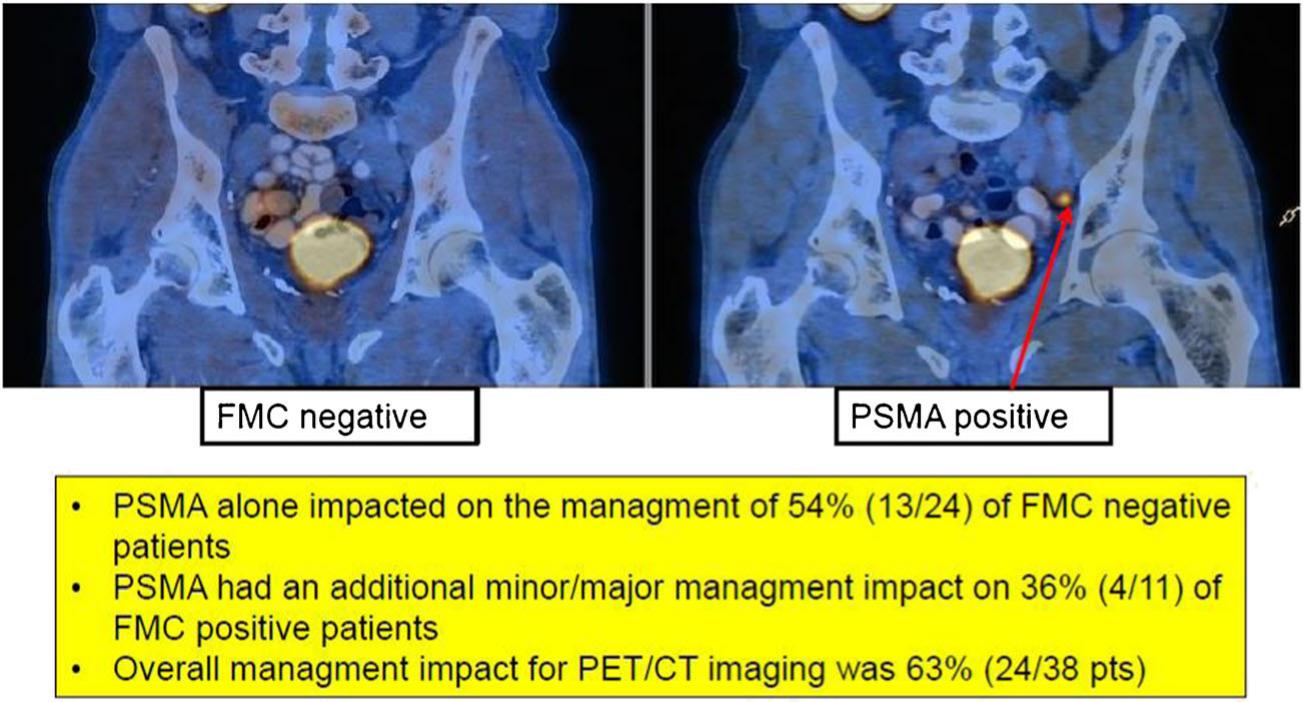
A variety of scientific presentations have shown superiority of PSMA-directed radiotracers as compared to choline-based PET tracers. Morighi et al. [2] have shown that PET sensitivity for detecting the anatomic site of biochemical relapse has doubled when ^68^Ga-PSMA is used as the PET radiotracer. The figure shows focally increased ^68^Ga-PSMA uptake in an iliac lymph node metastasis, whereas the corresponding ^18^F-Fluoromethylcholine-PET/CT scan showed no correlate of recurrent prostate cancer
